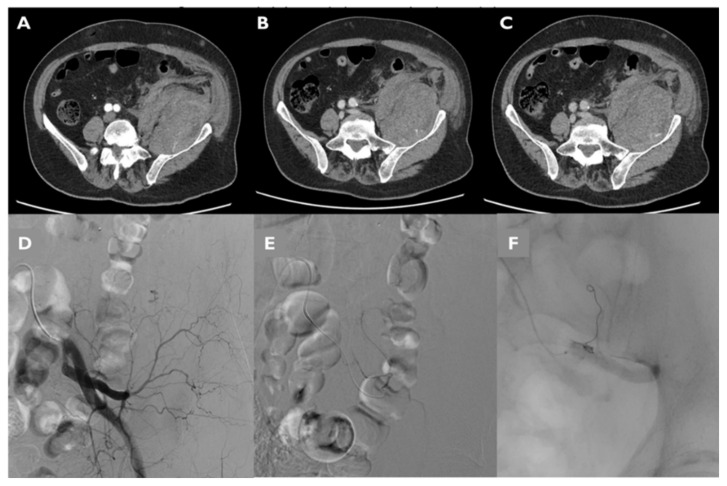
CT and DSA images of a patient presenting with a large hematoma of the iliopsoas muscle extending into the retroperitoneal space. Tri-phase contrast-enhanced axial CT images show active bleeding in the arterial (A), portal (B) and delayed phase (C). DSA shows active extravasation from left ILA (D). After super selective catheterization (E), PTAE was performed using a 3-mm coil and a gelatin sponge (F).
